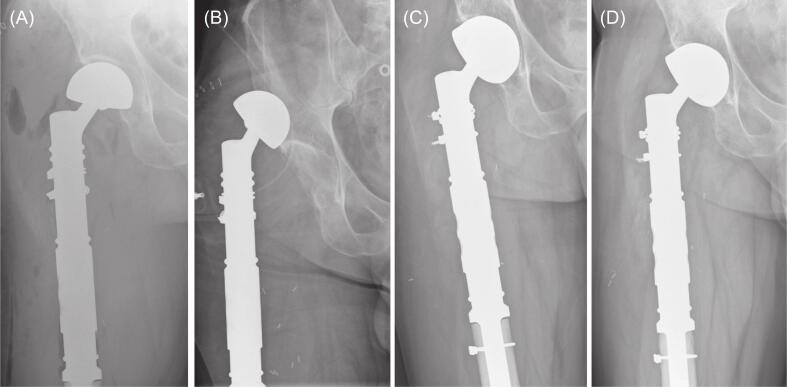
2 A: First postoperative radiograph after proximal femoral reconstruction with a MUTARS prosthesis in a 63-year old male patient with chondrosarcoma. 2B: Dislocation of the bipolar head nineteen days after surgery. 2C: Revision of the bipolar head to a dual-mobility cup. 2D: Two years after conversion to a dual-mobility cup, no complications reported.
